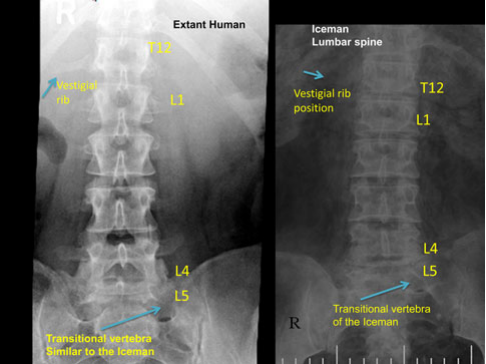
Iceman anteroposterior lumbar spine X-rays in comparison to an extant human with the same configuration of a right side thoracic 12 vestigial rib and lumbar 5 transitional vertebra. The Iceman’s vestigial rib is not as clearly seen in this image as in Fig. 6b
